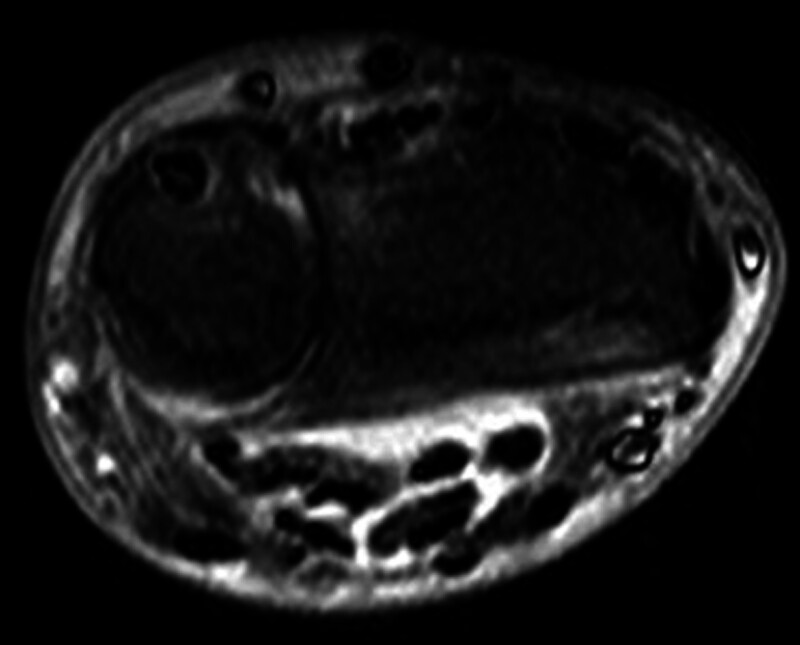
T2-weighted magnetic resonance imaging showing a high degree of synovial growth inside the carpal tunnel.
